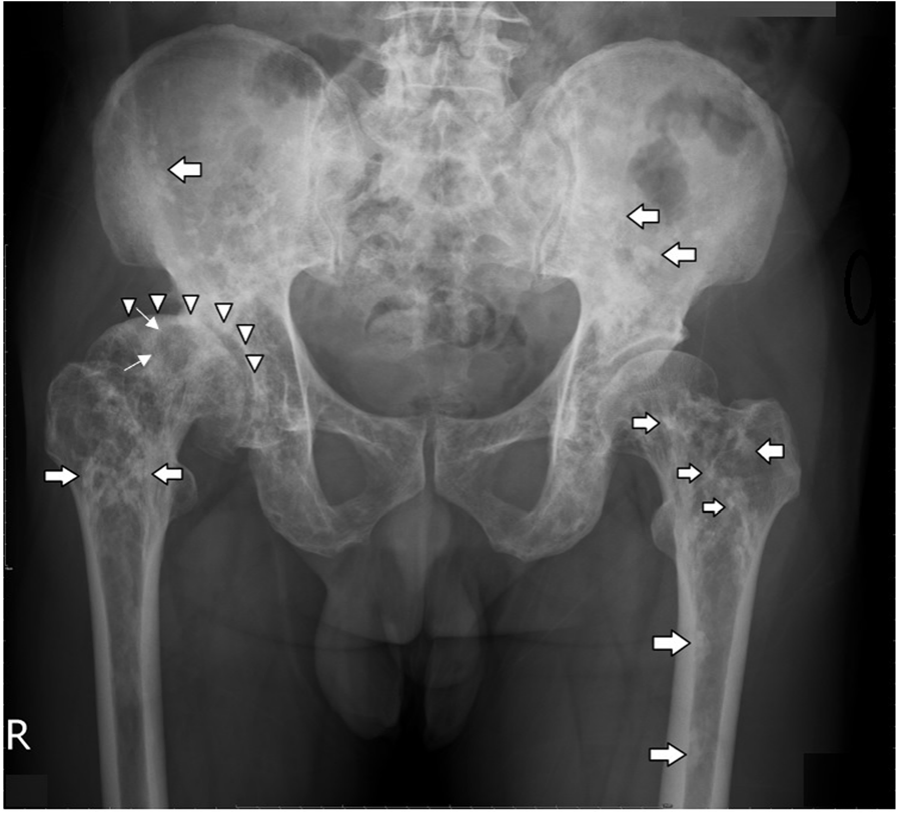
Plain radiography of pelvis. Multiple round and irregular radio-dense bone lesions in the pelvis and bilateral proximal femoral head (arrows). The right hip was typical of adult DDH, with shallow acetabulum and flattened femoral head (arrowheads). The presence of a subchondral cyst was also evident in the right femoral head. (angled arrow)
